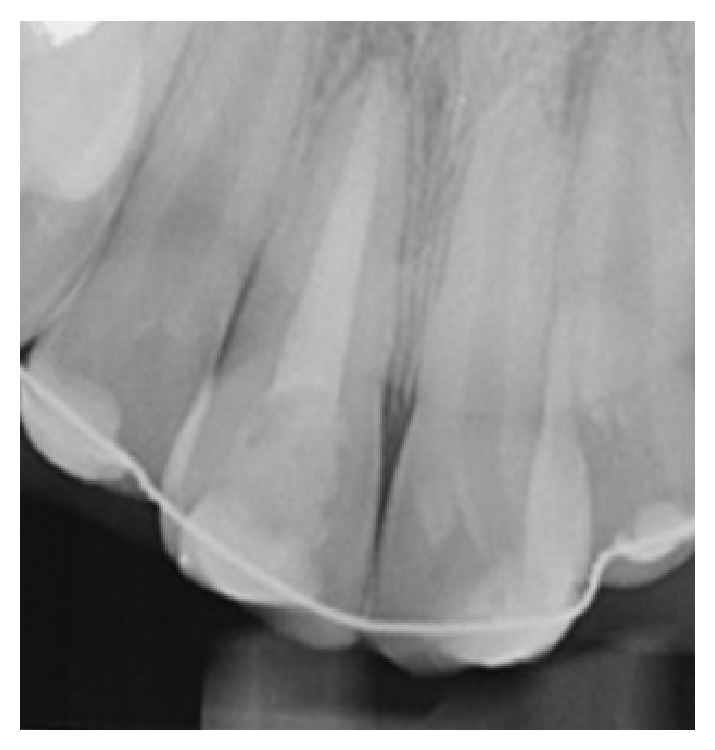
Periapical radiograph after replantation of avulsed tooth.
